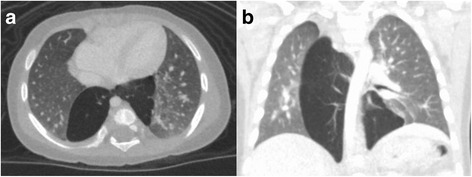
A chest computed tomography scan obtained from Patient 2 at the age of one month. A cystic mass in the left lower lung can be seen protruding into the posterior mediastinum and the opposite side of the chest (a. axial, b. coronal)
